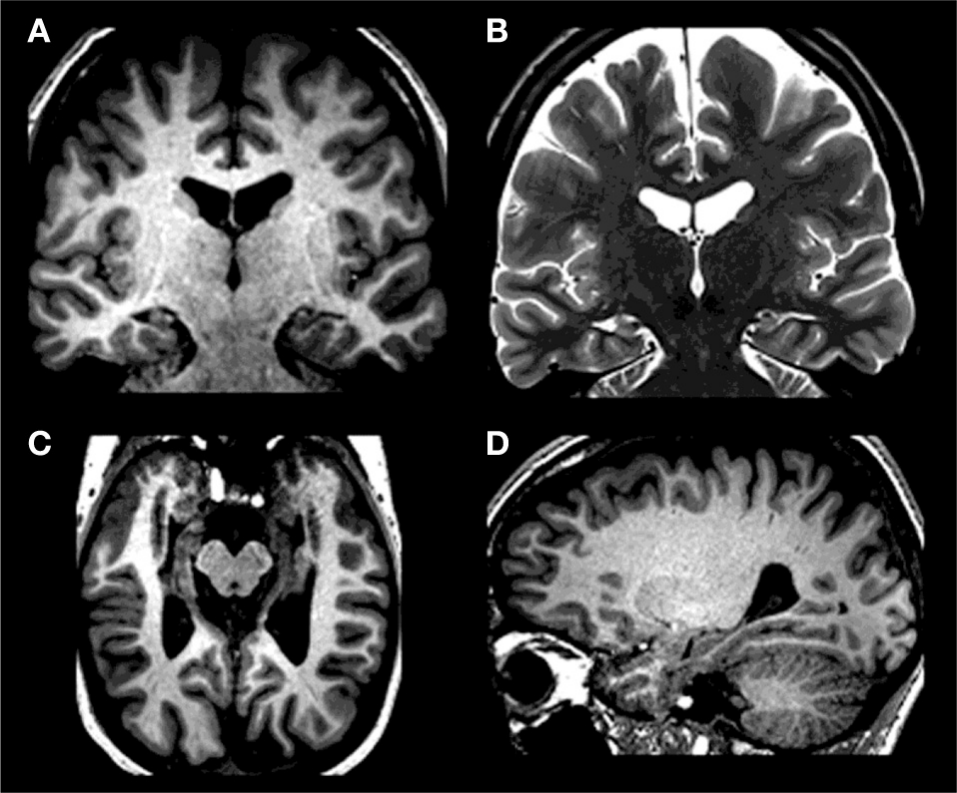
Bilateral hippocampal atrophy in ML with T1-weighted images [(A) coronal; (C) axial; (D) sagittal] demonstrating reduced hippocampal size in all directions – in the absence of marked extrahippocampal atrophy; T2 weighted coronal image (B) demonstrating bilateral loss of internal structure – here: a further marker of bilateral hippocampal atrophy. On clinical MRIs left side of the image is right side of the patient.
